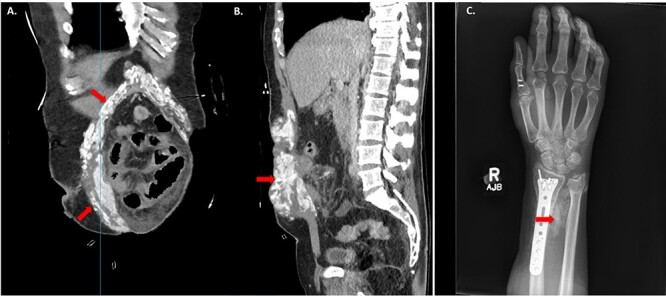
HO demonstrated on computed tomography (CT) imaging and X-ray after traumatic Injuries. (A) Coronal image of HO and (B) Sagittal image of HO on CT abdomen and pelvis demonstrating sites of HO (red arrows). (C) X-ray of right forearm between ulna and radius showing HO (red arrows).
